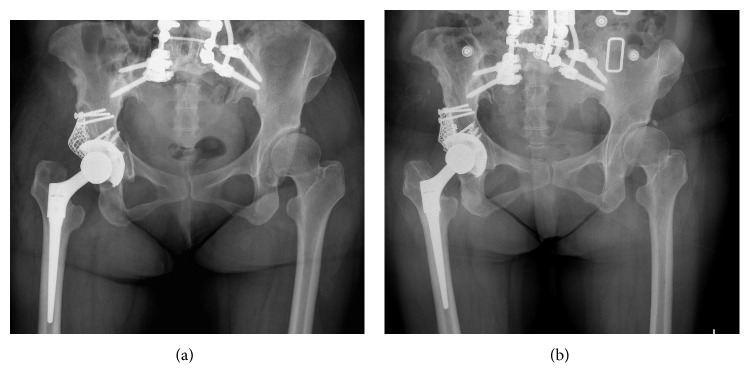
Case 1 postoperative hip X-rays. (a) Immediately after operation. (b) Nine years after operation. There were no clinical signs of implant loosening in the hip X-ray.
